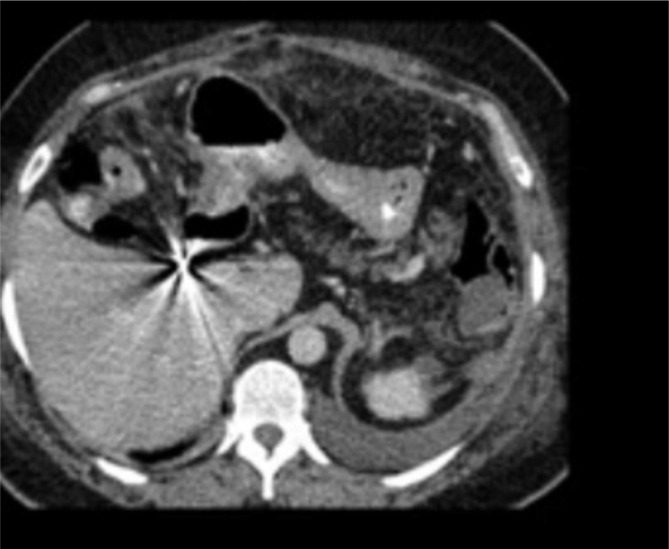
CT contrast post splenectomy after truma
